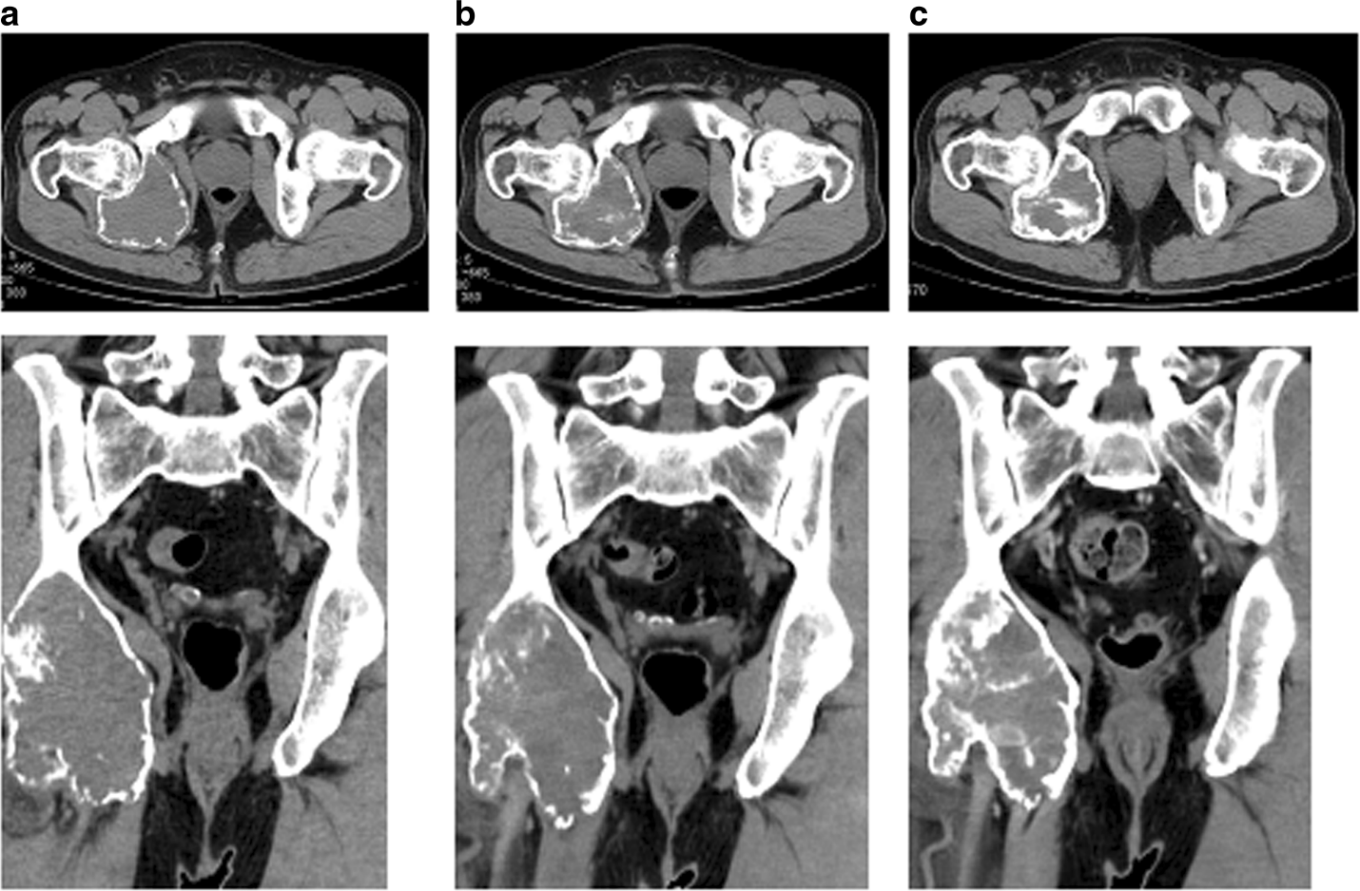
Analysis of pelvic CT images before administration (a), 1 month after denosumab administration (b) and 3 months after administration (c). Shell formation and cortex remodeling were observed at the tumor margin following denosumab administration.
